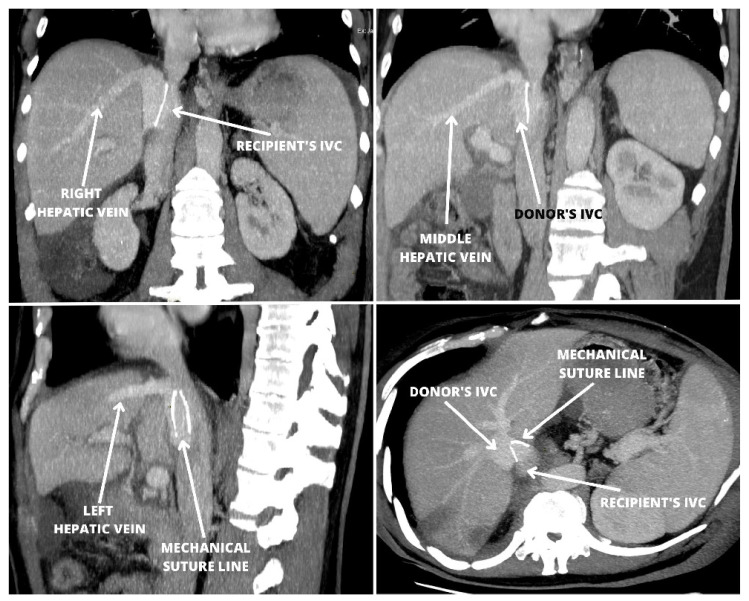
A follow-up computed tomography performed on the 12th postoperative day in the patient with stapled cavo-caval side-to-side anastomosis showed patent vascular anastomoses including anastomosis of the IVCs with no signs of hepatic venous obstruction. IVC—inferior vena cava.
